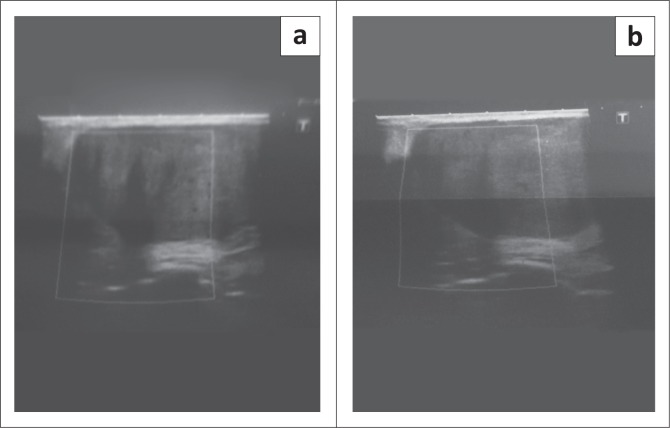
Longitudinal (a) and transverse (b) sonographic images of the predominantly isoechoic, palpable lump.
